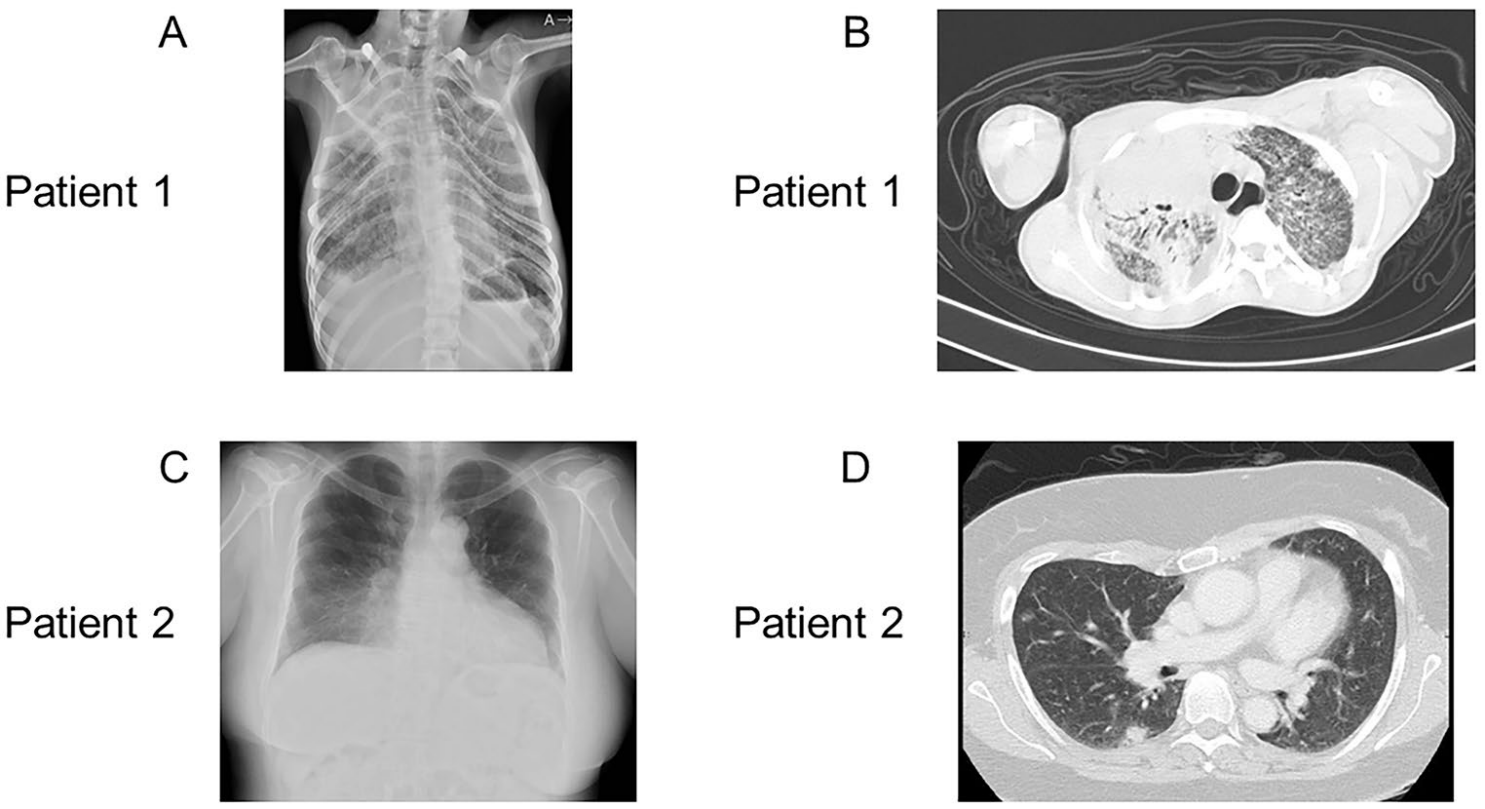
Radiological findings. A: (Patient 1) Chest X-ray revealing atelectasis in the right upper lobe. The right costophrenic angle is dull, with a granular shadow in the entire lung field. B: (Patient 1) Chest CT, showing slight compression of the right upper bronchus, presence of an air bronchogram in the right upper lobe, and consolidations in the left lung, which are believed to be inflammatory changes, suggesting that the patient has pneumonia. Granular shadows are observed in the lungs. C: (Patient 2) Chest X-ray showing abnormal bilateral lung shadows. D: (Patient 2) Chest CT showing mottled frosted shadows and irregular nodular shadows in both lungs
